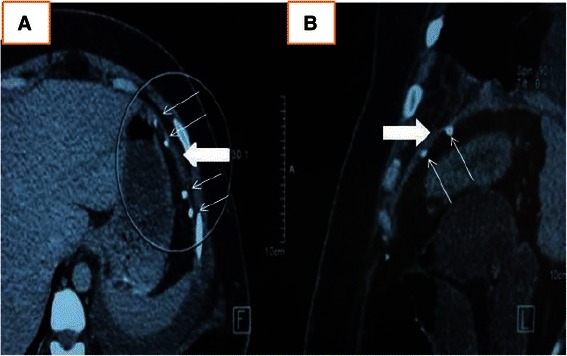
Axial (a) and sagittal (b) computed tomographic images showing continuity of the left hemidiaphragm (highlighted within the *circle*) reinforced with mesh (*thick arrows*) and ProTack (*narrow arrows*)
